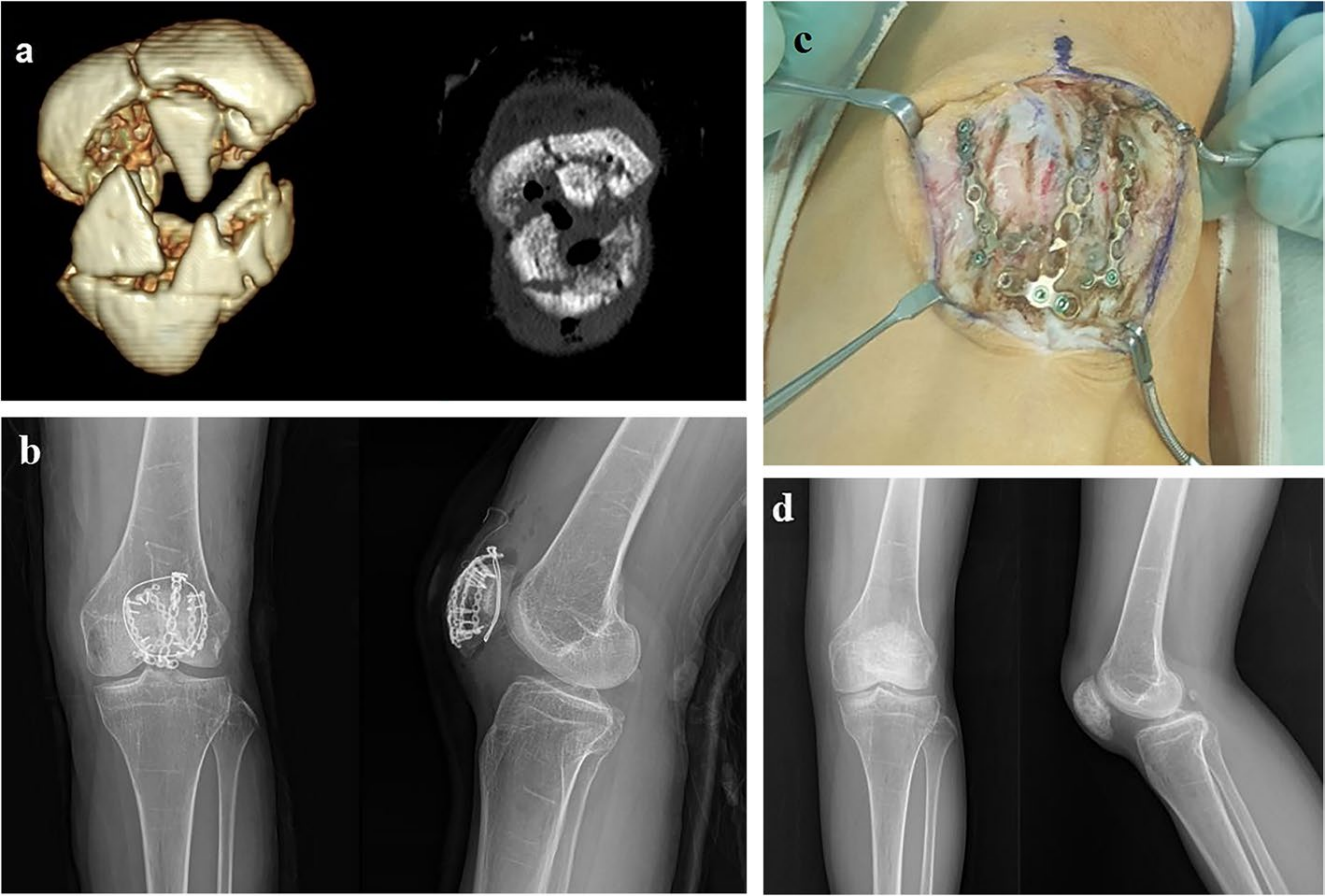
Preoperative and postoperative radiologic examination of Case 2 (Female, 52 years old) a preoperative CT coronal and 3D reconstructed image b postoperative simple radiograph AP and lateral view after internal fixation c intraoperative clinical photo of miniplates at the time of implant removal d postoperative simple radiograph AP and lateral view after implant removal
